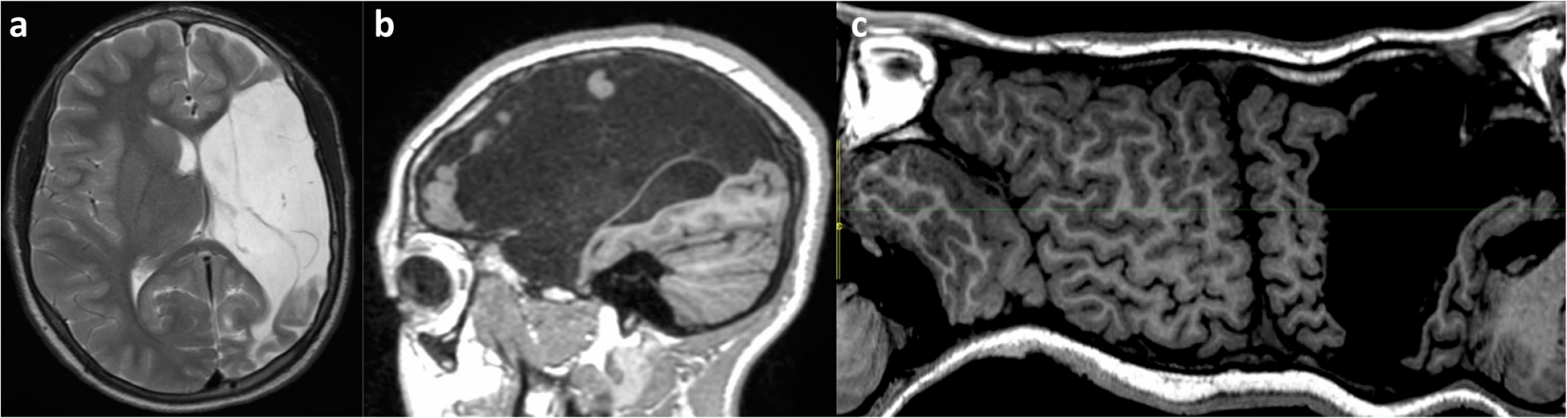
a–c MRI scan in a 14-year-old girl with a chronic left MCA infarct. Axial T2-weighted image (a), sagittal T1-weighted image (b) and Mercator map derived from the curved reconstruction of 3D T1 images (c) show the unilateral sharply demarcated cystic encephalomalacia involving the arterial territory of the left MCA. Notably, there is involvement of the left temporal lobe, the deep nuclei and the inferolateral aspect of the frontal lobe with sparing of the parasagittal region and superior frontal gyrus, i.e. the watershed. The involvement of the superior temporal lobe which is not a feature of parasagittal extension in severe partial-prolonged hypoxic-ischaemic injury in term neonates can be appreciated, especially on the sagittal image (b). The Mercator map derived from the curved reconstruction of 3D T1 images (c) confirms the unilateral left MCA territory cystic change and provides an overview comparing the left with the right side of the brain. It also shows that the inter-hemispheric fissure and superior frontal gyrus are relatively preserved (i.e. not expanded/separated through atrophy)
